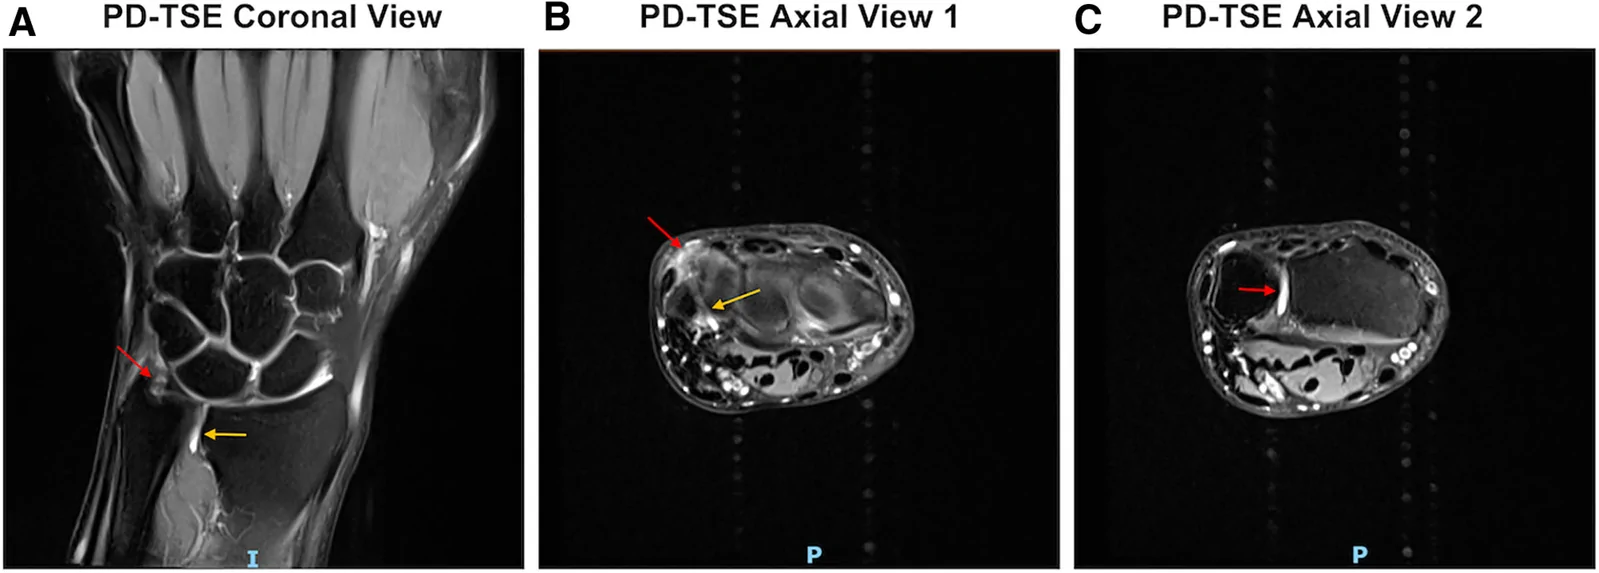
Magnetic resonance imaging of case 1: (A) coronal view demonstrating a long liner signal extending from the upper portion of the TFCC to the bottom, indicating an avulsion TFCC injury (red arrow). Besides, joint effusion was present within the DRUJ (orange arrow); (B) Axial view demonstrating long signals from dorsal to volar side ulnarly (red and orange arrow), consistent with the tear in the coronal view; (C) Joint effusion in DRUJ extending to the dorsal side (red arrow), indicating possible tear of dorsal ligament of the DRUJ. DRUJ = distal radioulnar joint, TFCC = triangular fibrocartilage complex.
